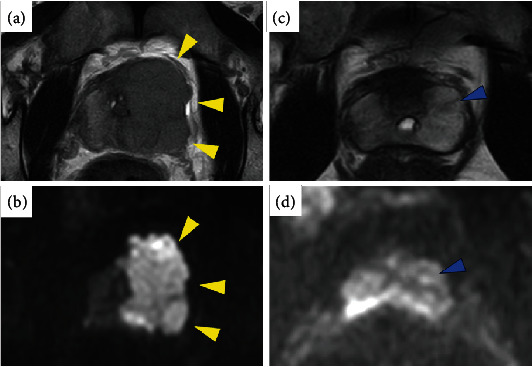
Pelvic magnetic resonance imaging: (a) axial T2-weighted image and (b) diffusion-weighted image revealed prostate tumor at diagnosis of metastasis. (c) Induction chemotherapy resulted in prominent shrinkage of the tumor and (d) obscuration of the high signal on diffusion-weighted image.
